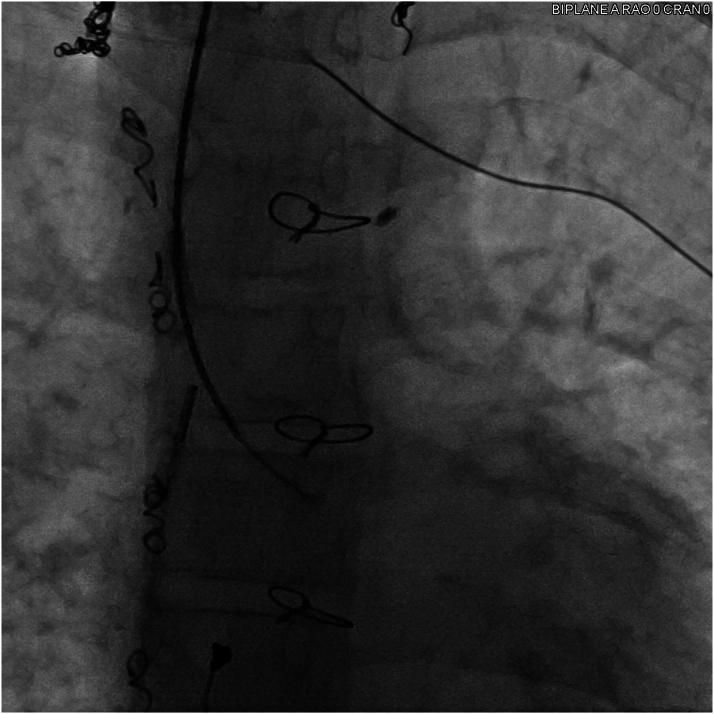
Fluoroscopy of Transpulmonary Puncture Trans-septal needle from the superior vena cava puncturing through the proximal left pulmonary artery to the epicardial space. The intracardiac echocardiography catheter is positioned from the right femoral vein across the Fontan baffle at the bifurcation of the pulmonary arteries, with the ultrasound vector directed at the trans-septal needle and transpulmonary puncture site.
